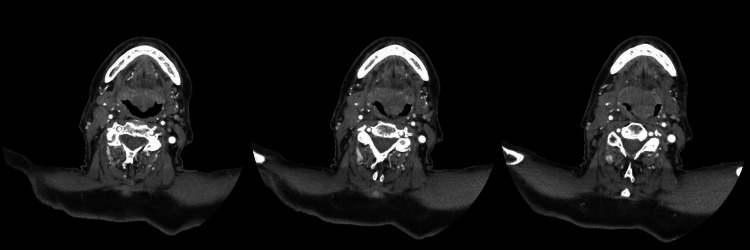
Case 1 CT scan of the neck showed swelling in the right median-paramedian site with homogeneous density, involving the lingual surface of the epiglottis and the root of the tongue. The glottic plane is asymmetrical, with thickening of the posterior and left lateral walls of the larynx in the supraglottic position CT: computed tomography
